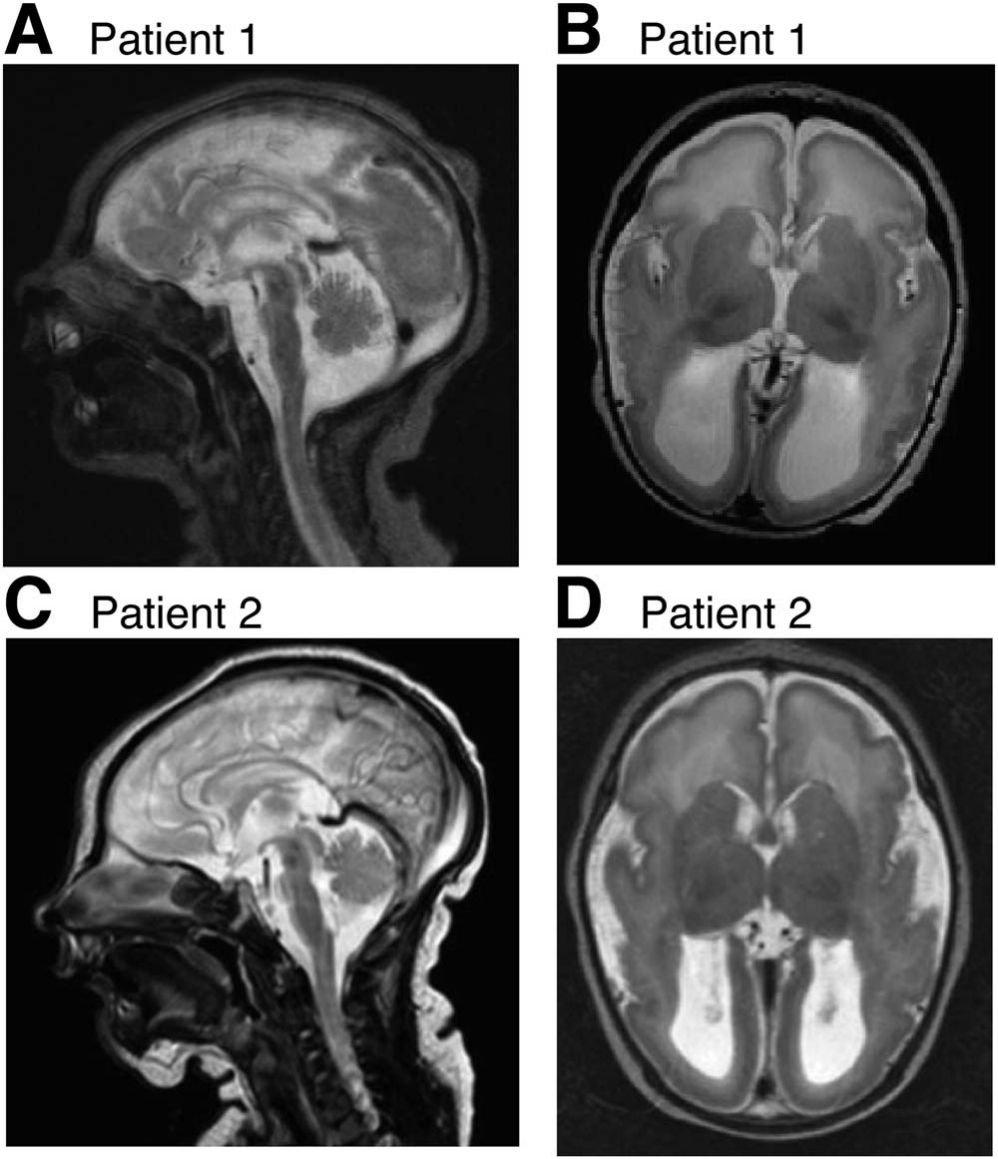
MRI images. (A and C) T2 weighted sagittal image shows a thin corpus callosum with hypoplastic splenium. It also shows hypoplastic pons and normal vermis. (B, D) T2 weighted axial image shows posteriorly enlarged ventricles with intraventricular cysts. There is temporo-parietal polymicrogyria, no cortical folding in the occipital lobes, and there is a simplified gyral pattern in the frontal lobes. Subcortical band heterotopia is seen posteriorly. The images were taken at the age of four days (patient 1) or five days (patient 2).
